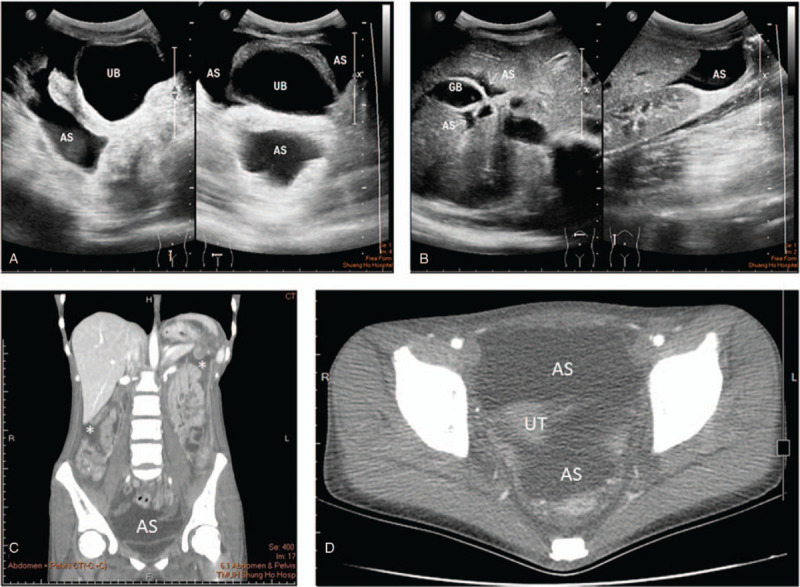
Abdominal sonography and CT scan showed diffuse ascites in the pelvis (AS in A and C: coronal section), Morison pouch, and subsplenic space (AS in B and asterisks in D: transverse section). AS = ascites, GB = gallbladder, UB = urinary bladder, UT = uterus.
